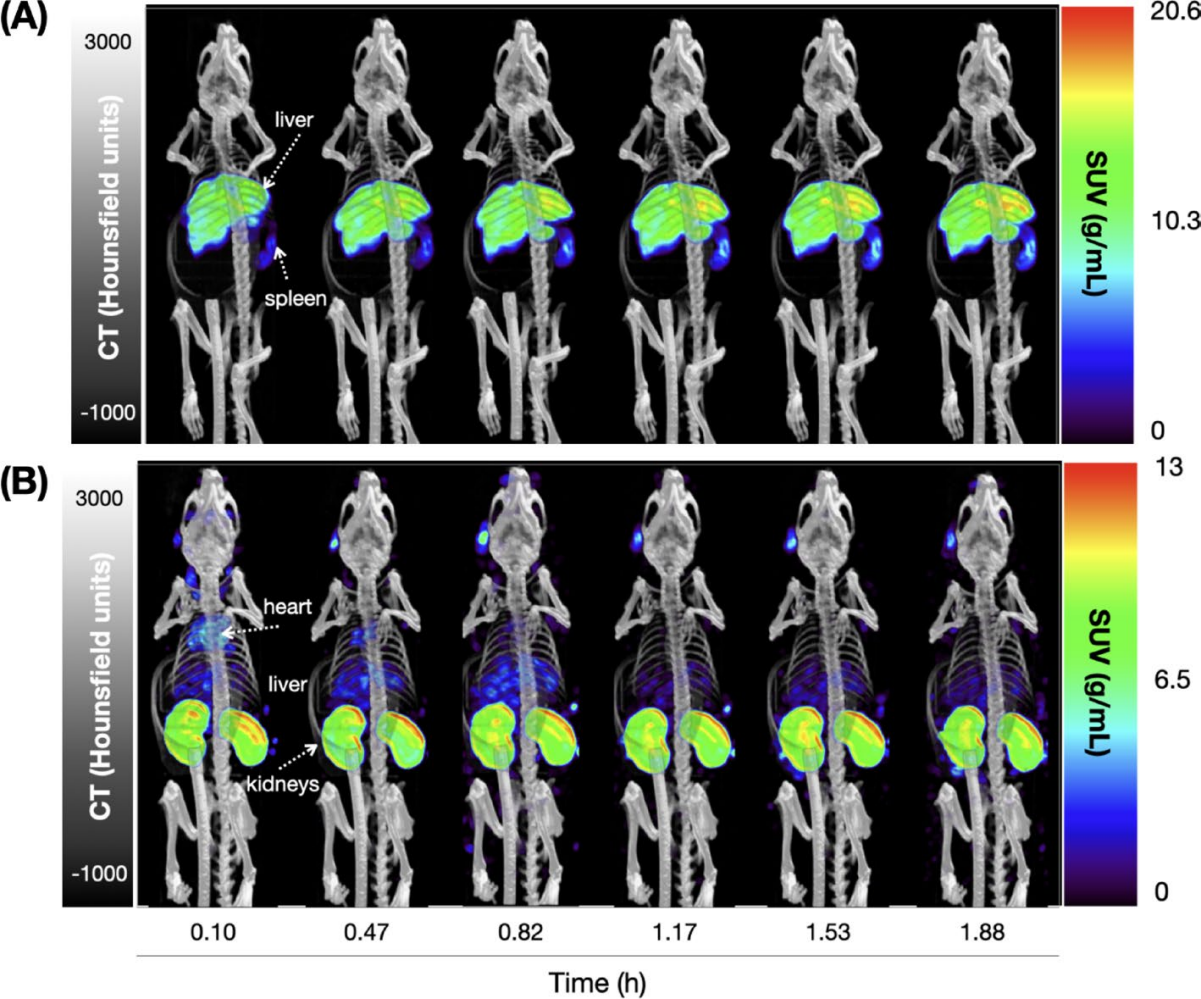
Maximum intensity projection (MIP) images depicting frontal view from a total body SPECT/CT scan obtained 0–2 h following the administration of (A) [^197m/g^Hg]Hg-Tetrathiol (0.899 MBq ^197m^Hg + 4.230 MBq ^197g^Hg), with comparison to the total body SPECT/CT scan obtained 2 h following the administration of (B) [^197m/g^Hg]HgCl_2_ (0.492 MBq ^197m^Hg + 3.171 MBq ^197g^Hg). Images reconstructed using the 77 keV photopeak
